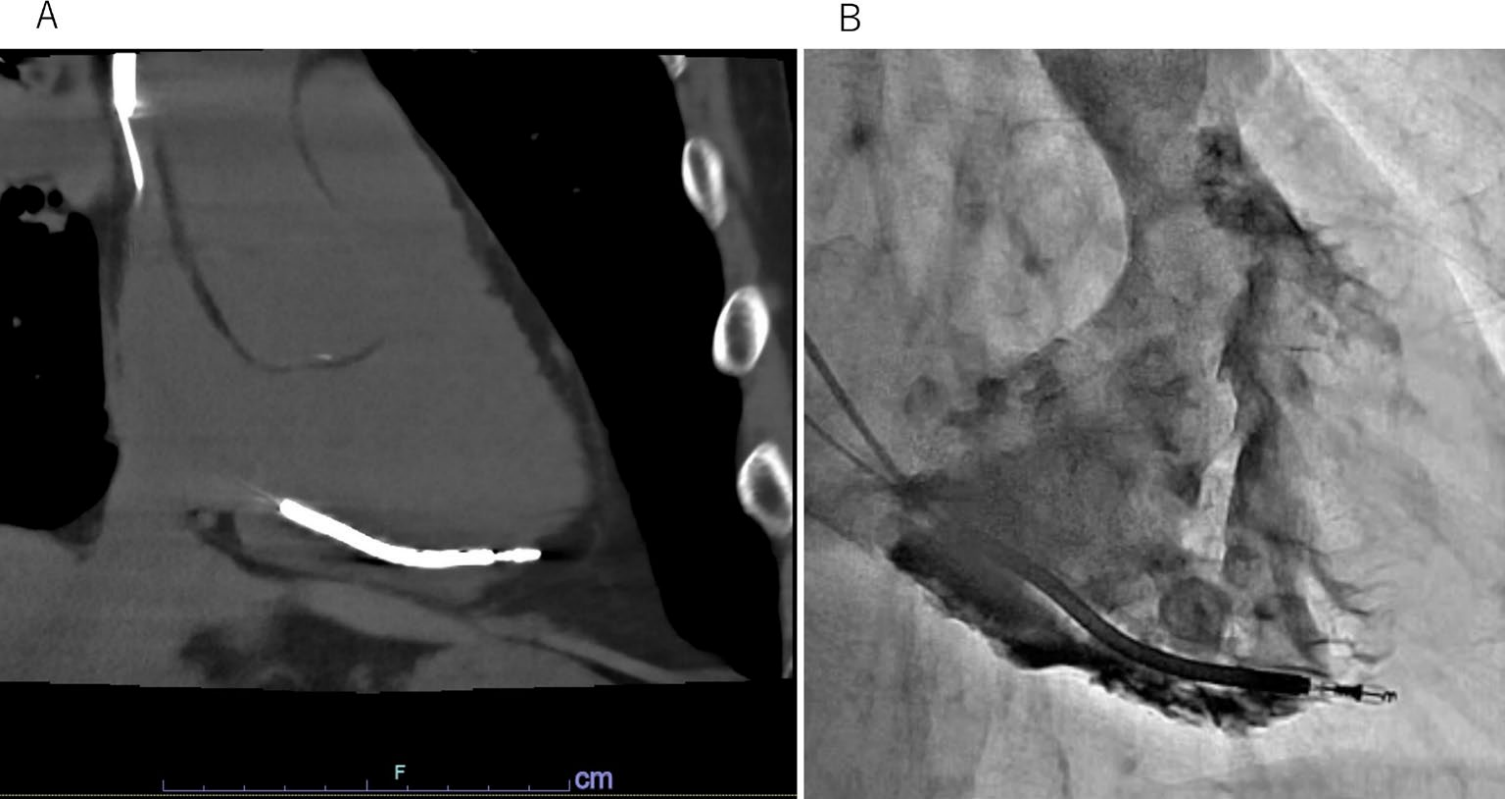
Computed tomography (A) and right ventriculography (B) suggest right ventricular (RV) lead‐tip perforation beyond the myocardial border.
